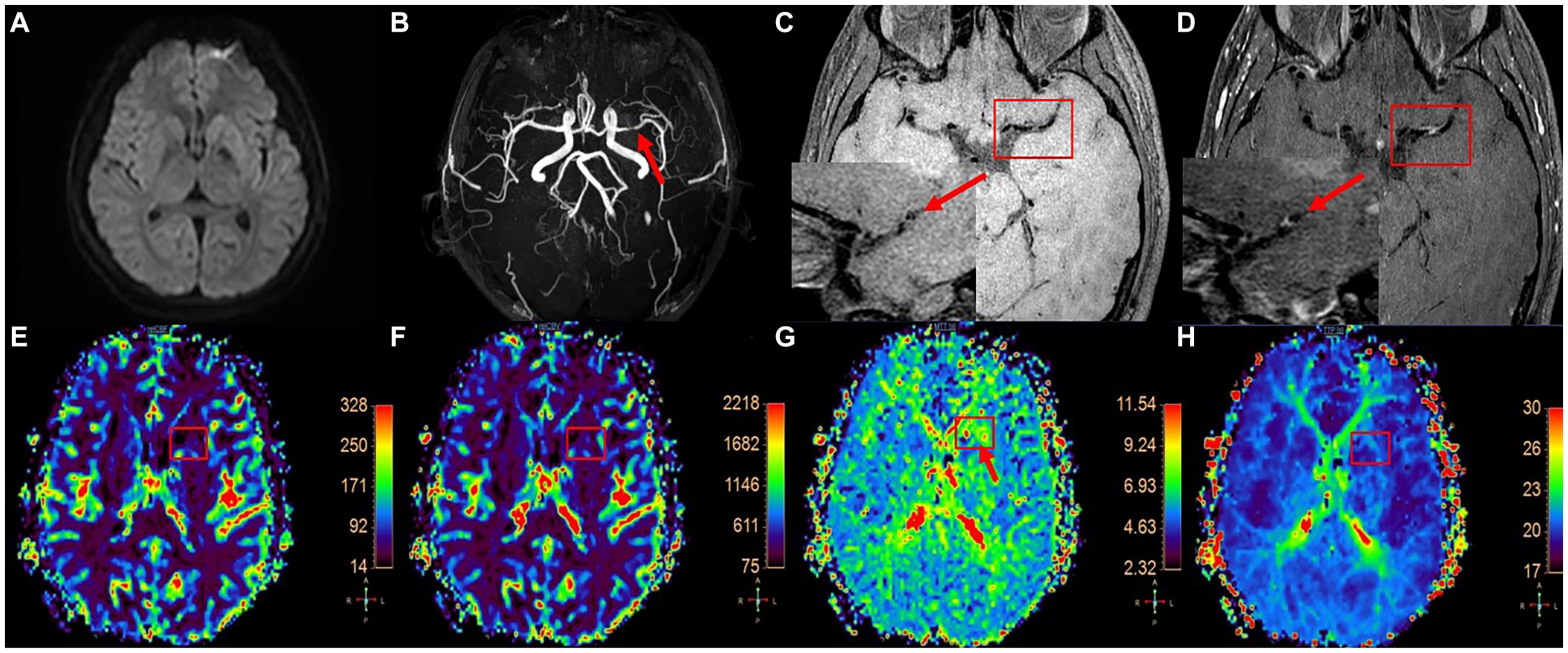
A patient with left MCA plaque was admitted to the hospital because of weakness in the right lower limb and was clinically diagnosed with anterior circulation TIA. DWI image shows that there is no diffusion restriction in the blood supply area of left MCA (A), 3D-TOF-MRA shows local stenosis of left MCA (B, as shown by the arrow), T1W-VISTA image (C) shows thickening of MCA wall (short axis view of MCA in the lower left corner, as shown by the arrow) and plaque formation, and obvious enhancement can be seen in enhanced scanning (D). DSC-PWI pseudocolor image (E–H) shows abnormal perfusion in the right basal ganglia.
